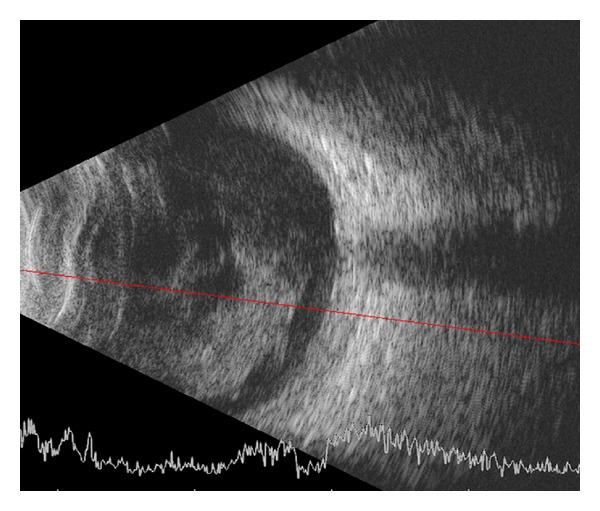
B-scan ultrasound demonstrating no signs of retinal detachment or obvious intraocular foreign body.
